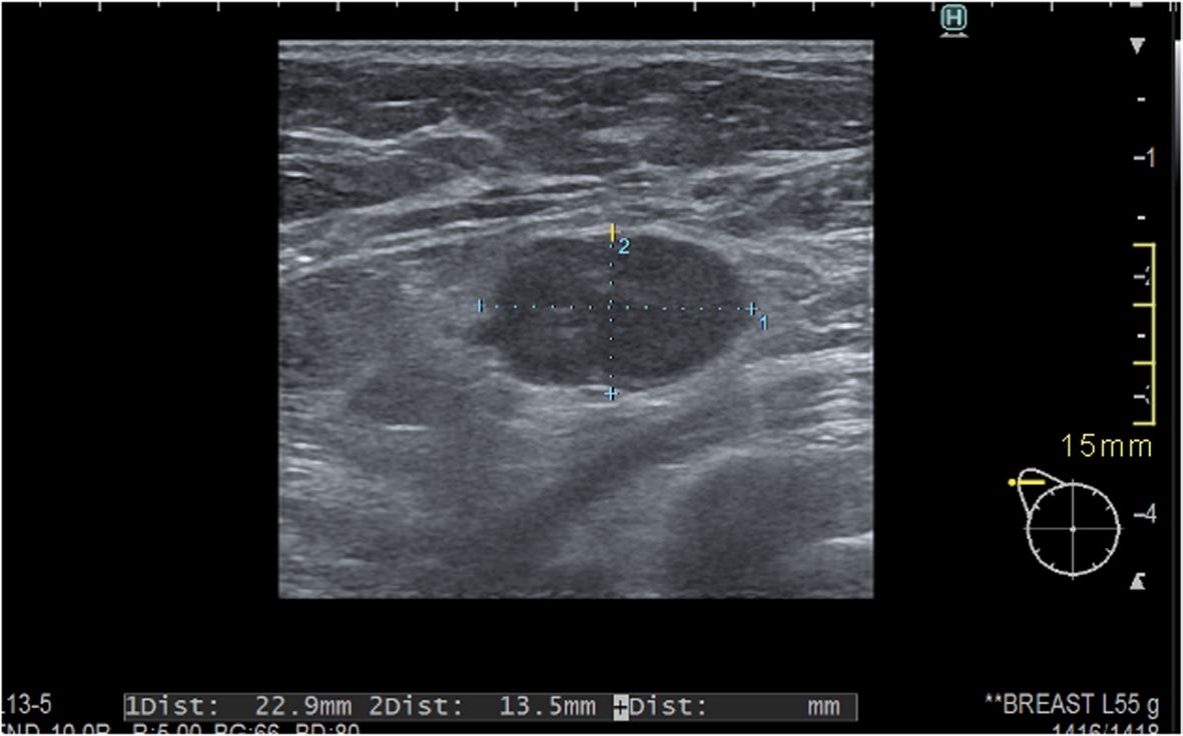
Persistent pathologic axillary lymph node on ultrasound after neoadjuvant systemic treatment measuring 23 × 13.5 mm
